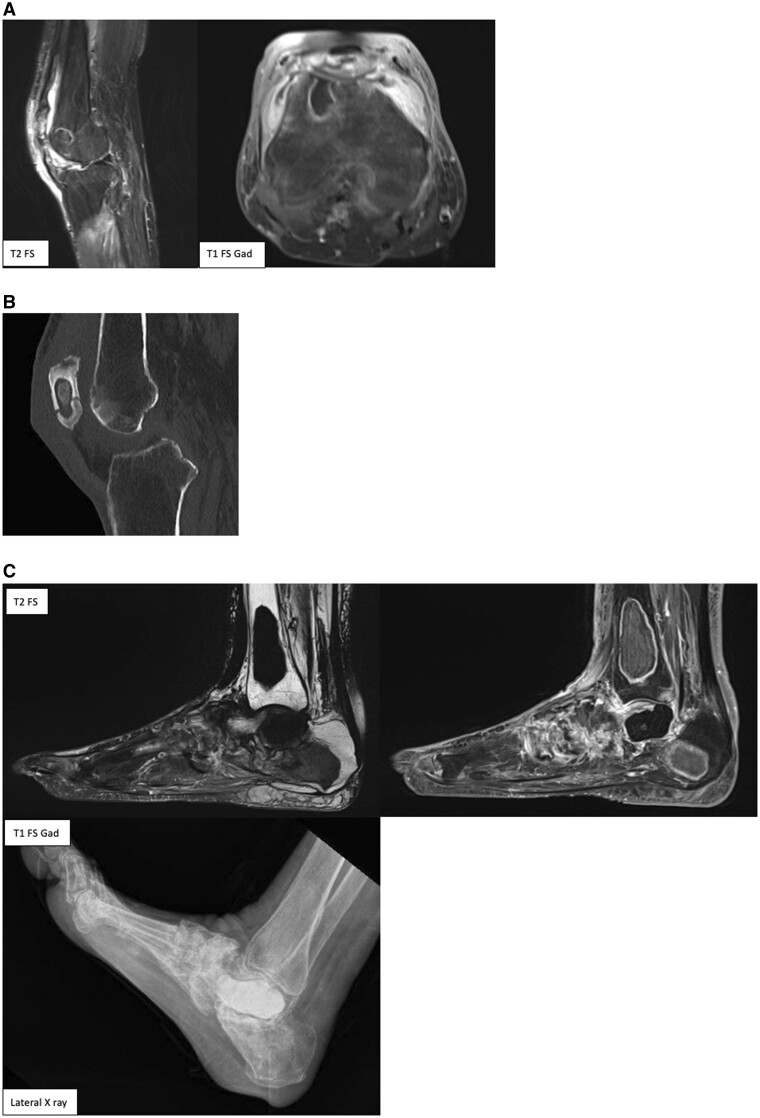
(A) Intramedullary fat necrosis with formation of cystic spaces in the distal femur and patella. Intramedullary lesions demonstrating T1- and T2-weighted low signal in the distal femur and patella. There is surrounding rim of enhancement. This is consistent with intramedullary fat necrosis. (B) Pathological fracture of the patella associated with intramedullary fat necrosis. Minimally displaced fracture line through the patella. There is a cystic space within the body of the patella which contains subtle calcification. (C) Intramedullary fat necrosis with cystic spaces and bone cement. Multiple lesions demonstrating low signal in T1- and T2-weighted images. There is partial collapse of the talus, which is filled with bone cement with very low signal T1 and T2 signal, this is seen with increased attenuation on radiograph.
